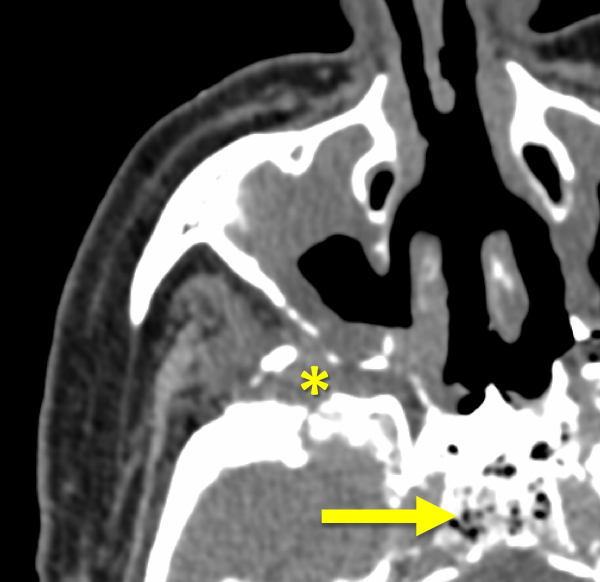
Computed Tomography of Paranasal Sinuses (Axial Plane, Soft-tissue Window). There is content in the right maxillar sinus with periantral invasion (asterisk). Air bubbles can be seen in the sphenoid bone (arrow).
